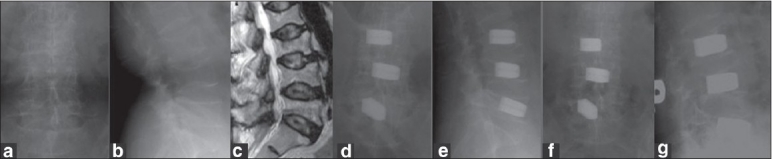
(a-c) Radiograph (anteroposterior and lateral) and T2W sagittal MRI in a 62-years-old female who suffered severe low back pain and was unable to upright her back without root signs showed L3–L5 osteoporotic compression fractures without root compression. (d,e) Radiograph (anteroposterior and lateral view) revealed three-level TpBA vertebroplasties without laminectomy immediately after operation. (f,g) Radiograph (anteroposterior and lateral views) at 4-year follow-up demonstrated good status of TpBA vertebroplasties
